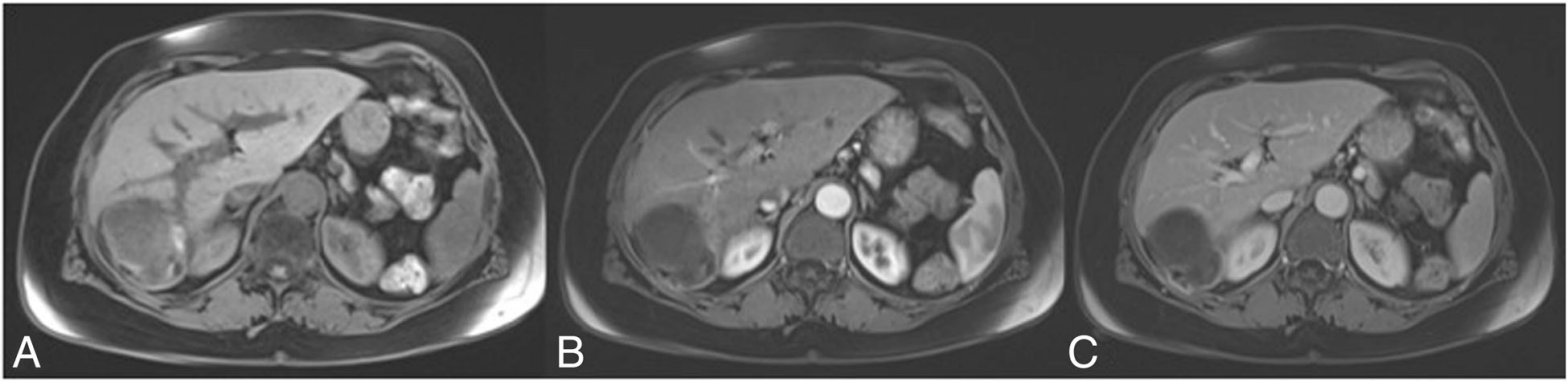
FS-T1-weighted pre (a) and post intravenous contrast injection (b, arterial phase and c, venous phase) MR images confirmed the hydatid hepatic cyst at the VII segment
